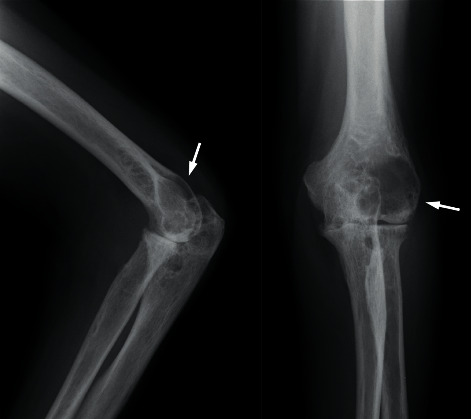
Plain radiograph of the left elbow obtained at the first visit to our department. A large transparent area is observed in the posterior lateral humeral bone (arrow). The vertical range is 29.8 mm, and lateral range is 33.0 mm in the anteroposterior view.
